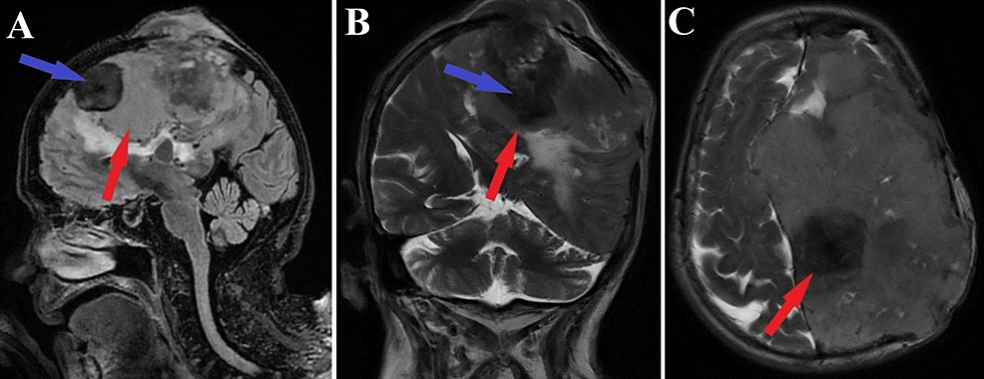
Preoperative MRI T2 and T2 FLAIR sequences Sagittal section of T2 sequence (A), coronal section of T2 FLAIR sequence (B), axial section of T2 FLAIR sequence (C). Red arrows indicate the giant malignant meningioma with various morphological aspects, while blue arrows highlight a calcification lesion. FLAIR: fluid-attenuated inversion recovery
